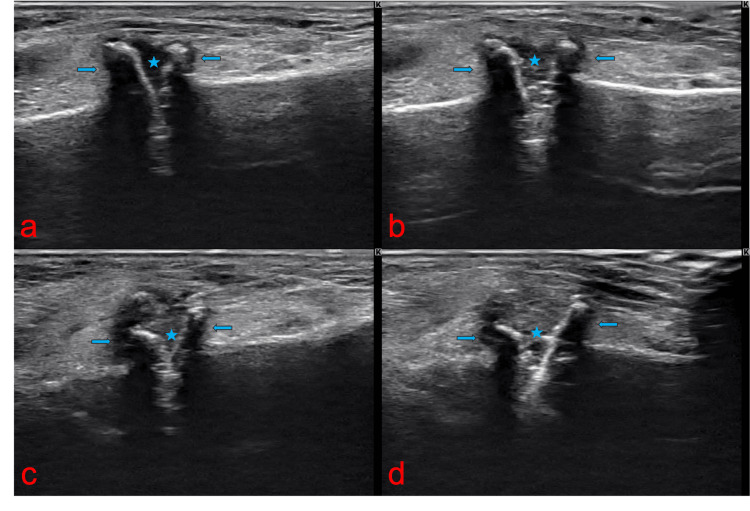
Ultrasound investigation The stars indicate the joint spaces, while the arrows highlight the osteophytes. (a) Neutral position of the second TMT joint showing joint space opening dorsally; (b) stress study in plantar flexion of the second TMT joint, revealing a wider joint space compared to the neutral position; (c) neutral position of the third TMT joint, similarly exhibiting joint space opening dorsally; (d) stress study in plantar flexion of the third TMT joint, demonstrating a wider joint space than in the neutral position. Following the excision of the osteophytes, there was significant concern about the potential for further joint instability.
